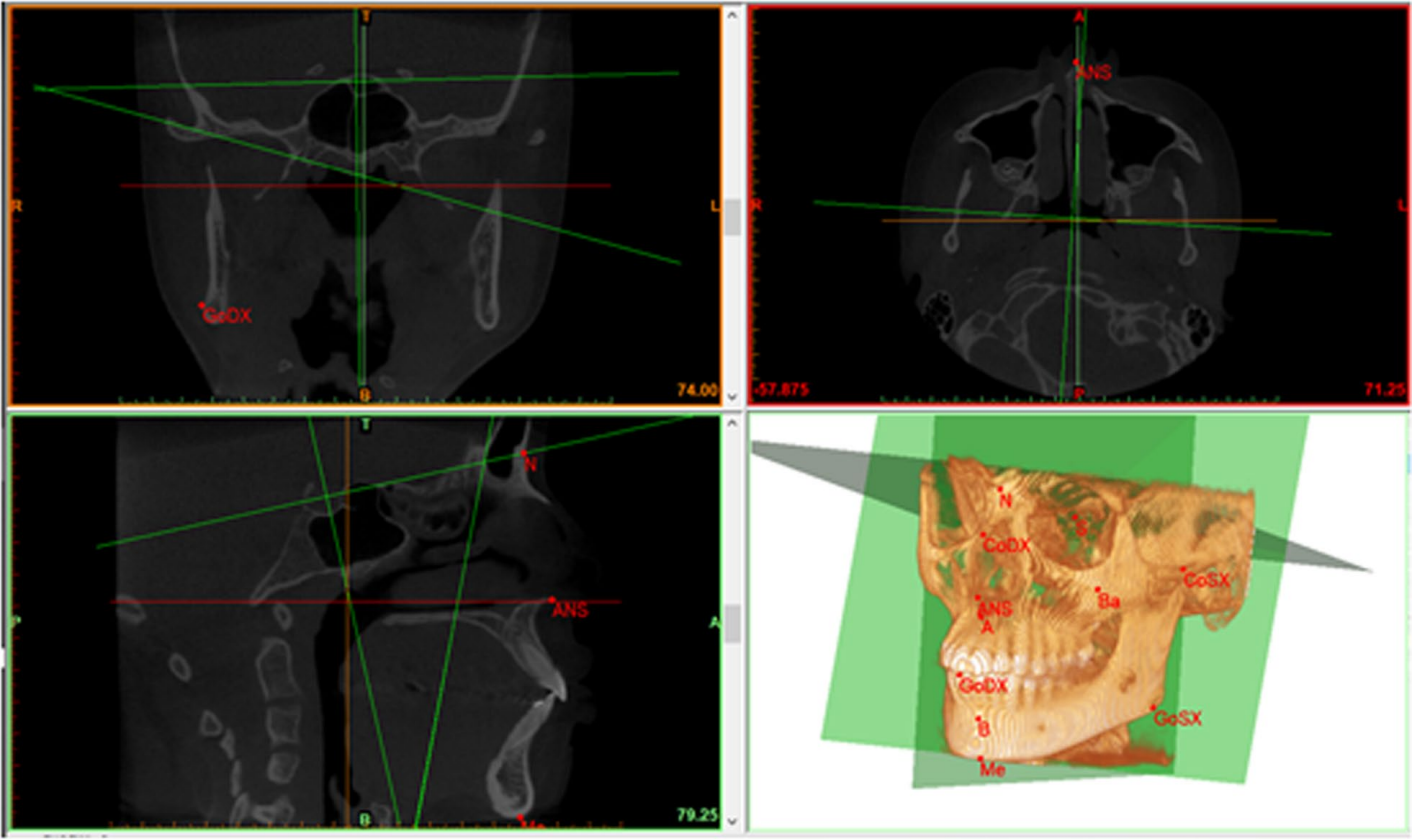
Cephalometric tracing of a CBCT on Materialise Mimics. At the bottom right some cephalometric points and all the three reference planes are visible
